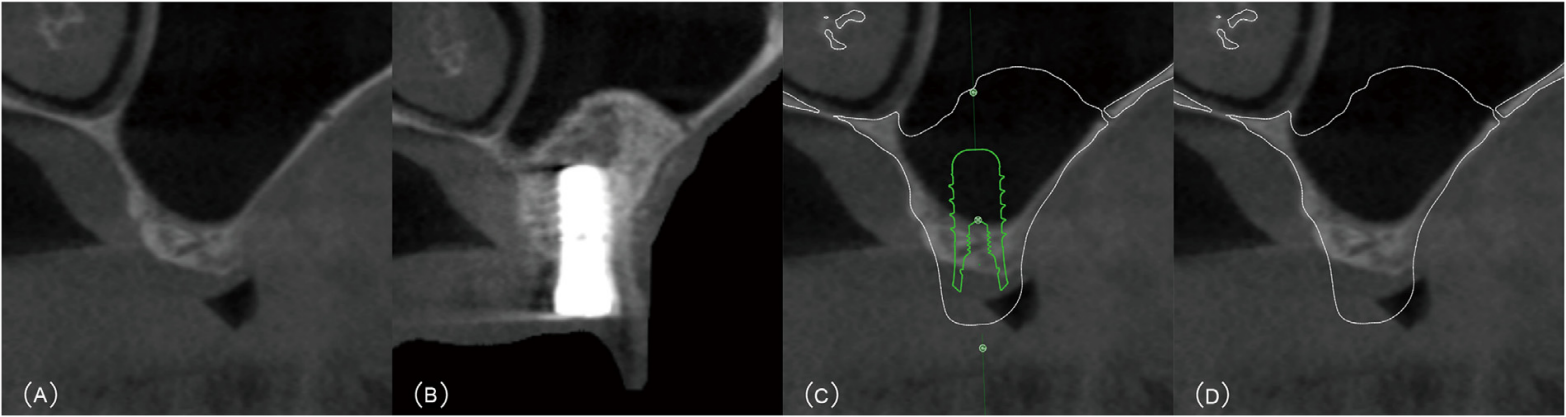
Determination of the assessment region on the CBCT image. A, Sagittal section of the preoperative CBCT showing the posterior maxillary ridge. B, Sagittal section of the postoperative CBCT showing the posterior maxillary ridge after implant placement and MSFA. C, Information on the implant, grafting bone and maxillary jaw was extracted from the postoperative CBCT data and then superimposed with the preoperative CBCT. D, The virtual implant was hidden to clearly expose the region for assessment.
